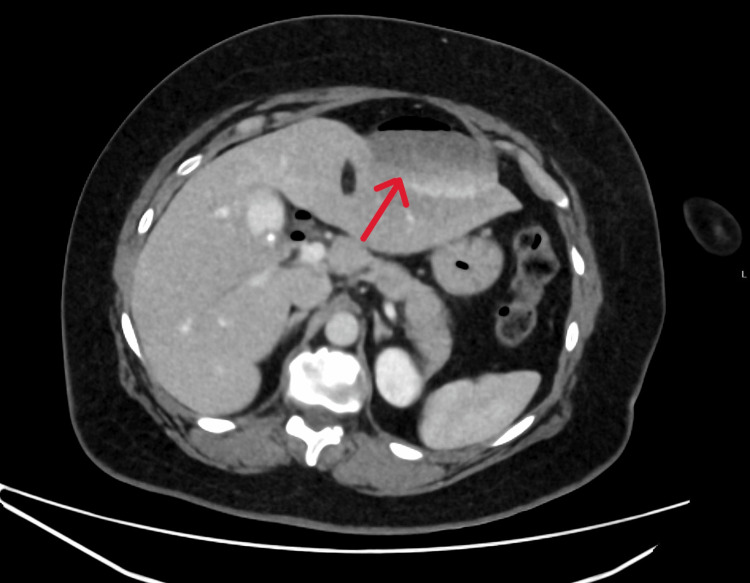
CT image showing a biloma
